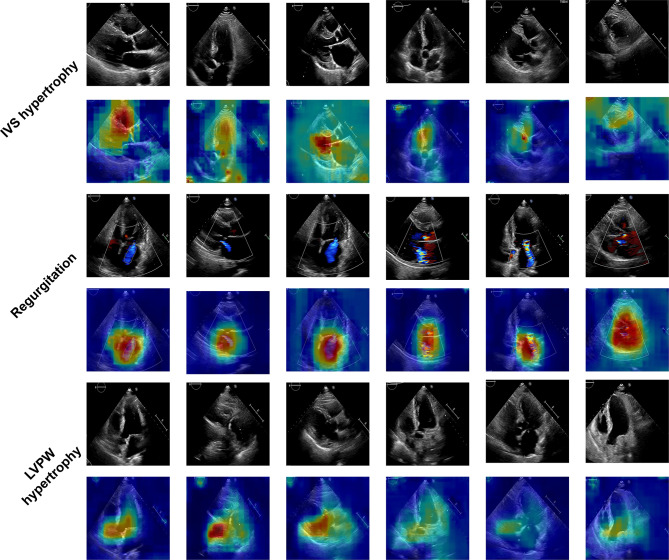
Model attention heatmaps on echocardiographic images for stroke risk prediction. This figure visually demonstrates the regions of highest model attention when assessing stroke risk. Rows 1, 3, and 5 display original echocardiographic images (B-mode and Color Doppler). Rows 2, 4, and 6 show the corresponding images with overlaid Grad-CAM heatmaps, where warm colors (red/orange) indicate high attention and cool colors (blue/green) indicate lower attention. The heatmaps consistently highlight critical cardiac features associated with stroke risk, such as interventricular septal thickening, left ventricular posterior wall thickening, left atrial chamber morphology, and valvular regurgitation
